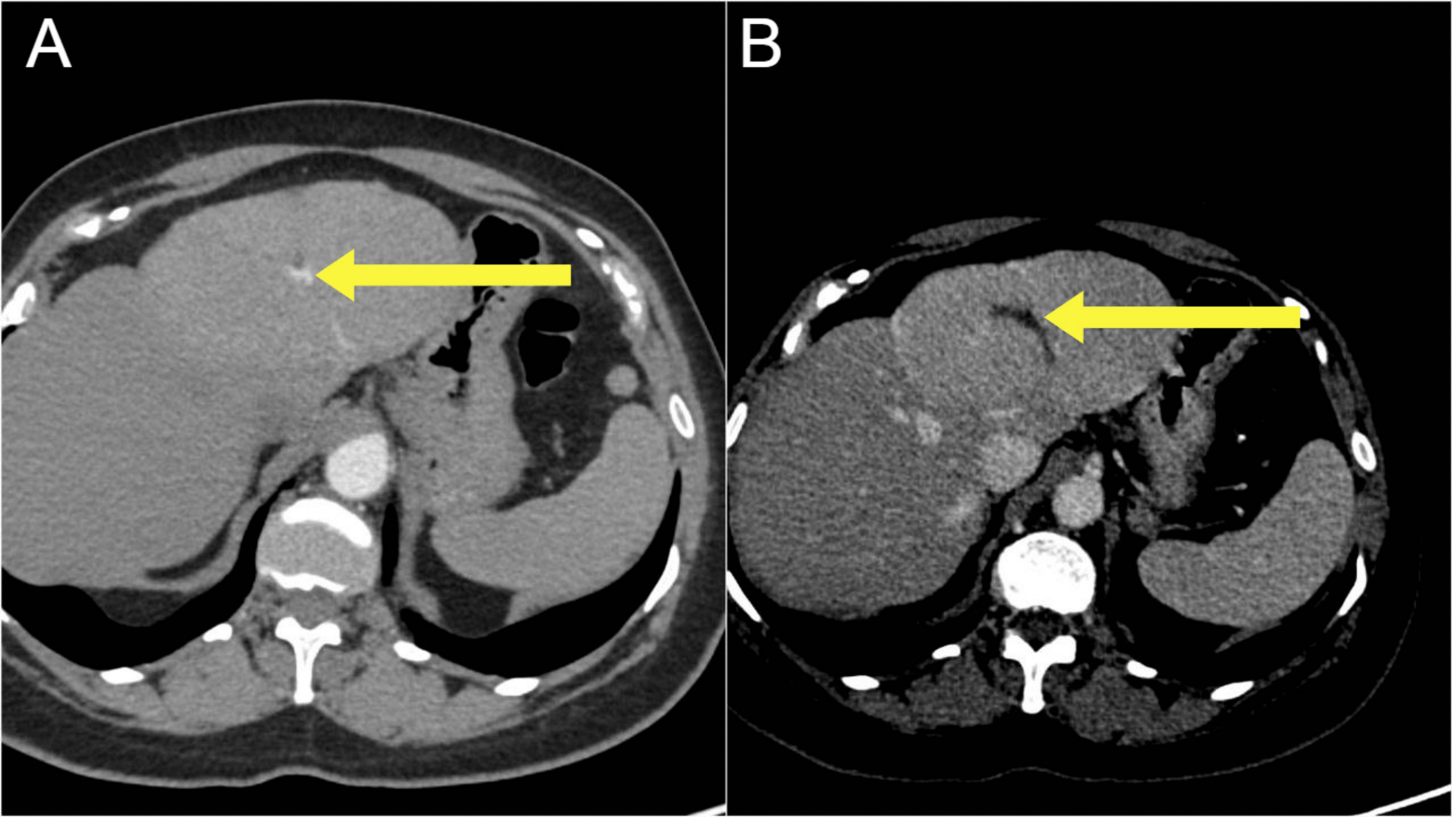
(A) Arterial-phase CT showing the feeding vessel in the center of the mass. (B) CT porto-venous liver window showing hyperattenuation of the mass compared to the normal liver parenchyma and the presence of a central scar.
